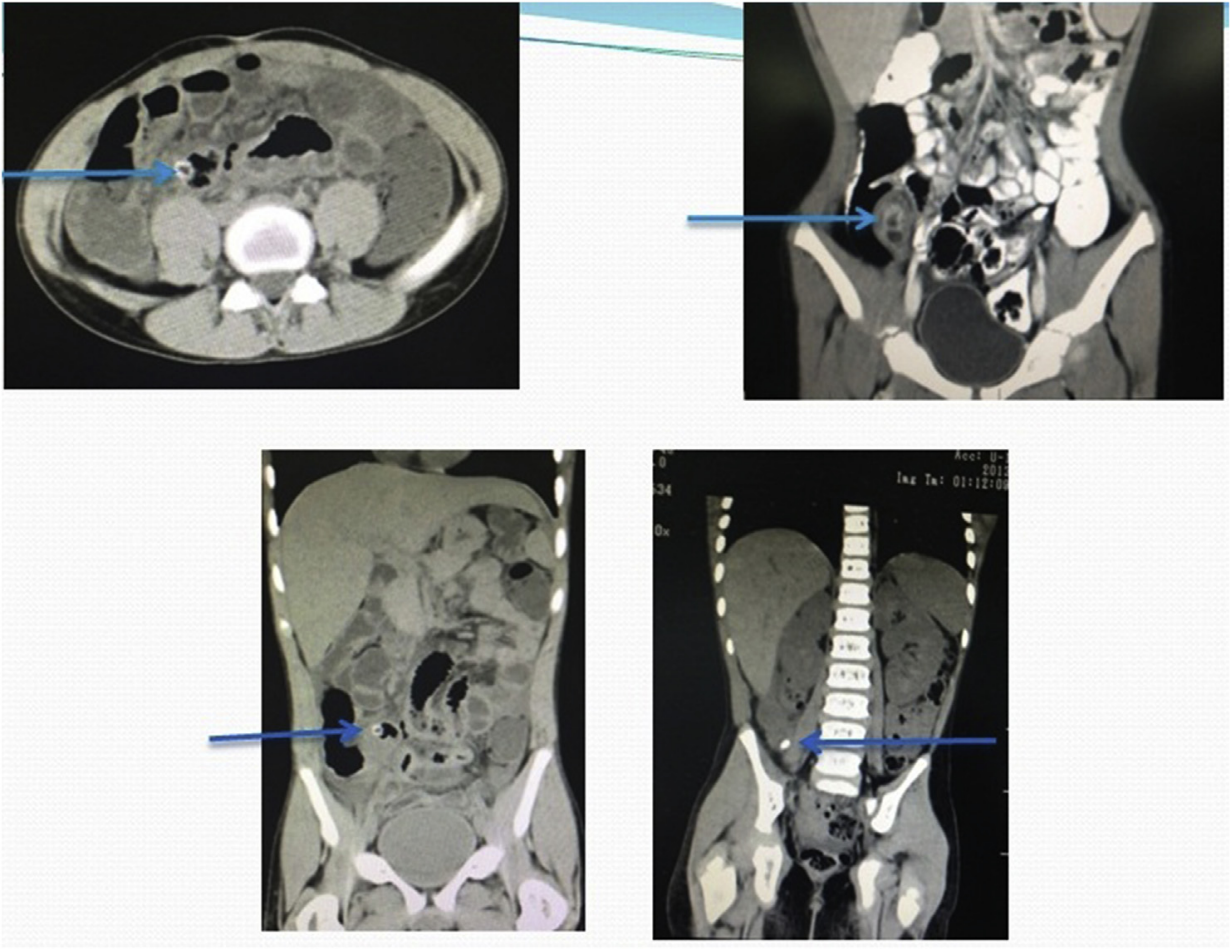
CT images show fecalith and acute appendicitis in equivocal cases (arrows).
